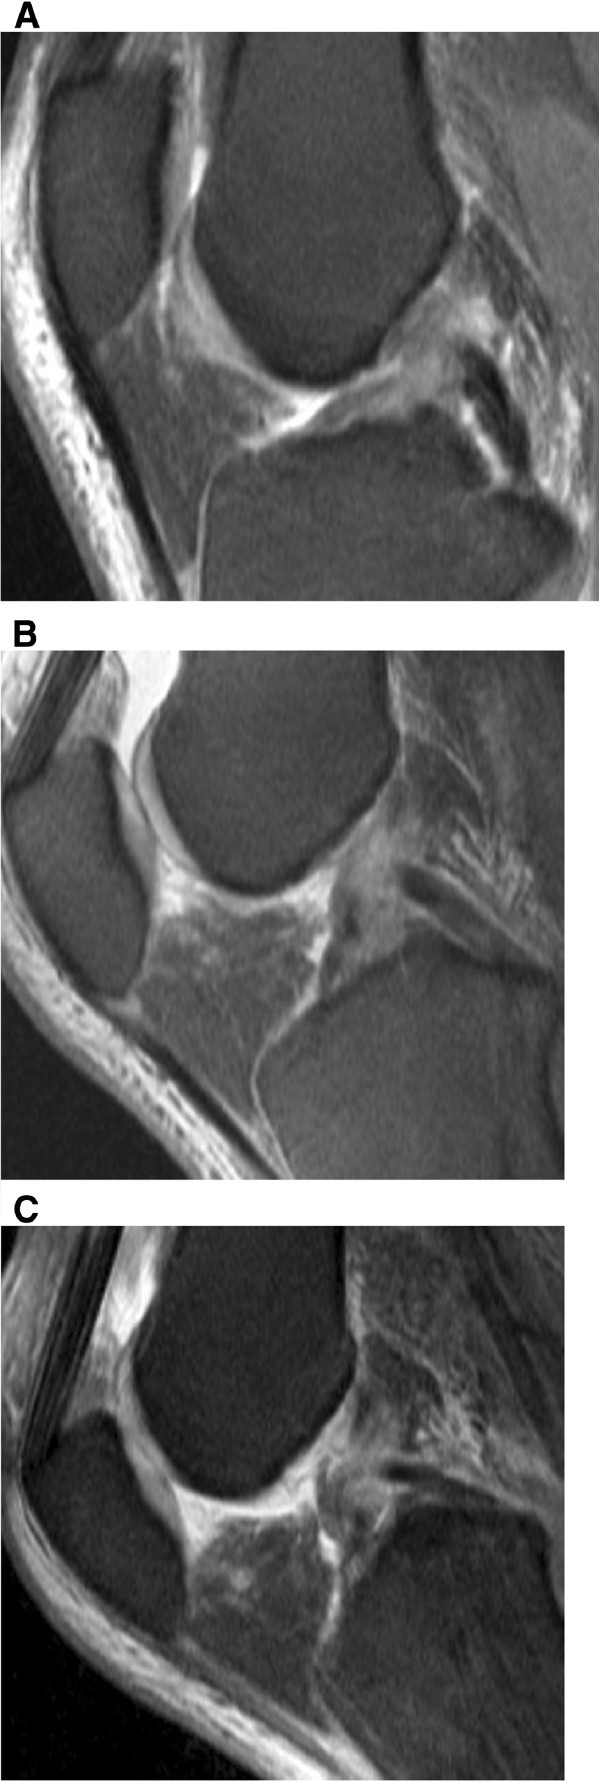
Arthroscopically proven partial ACL-rupture in a 53-year old patient after ski accident. (A) T2-weighted TSE FS sagittal image (2900/90) obtained at knee extension shows an irregularity of the midportion of the ACL. On T2-weighted TSE FS sagittal images (2900/90) at 30° of knee flexion (B) and at 55° of knee flexion (C) the partial continuity of the ACL bundles are better recognized than on MR images taken at knee extension (A).
